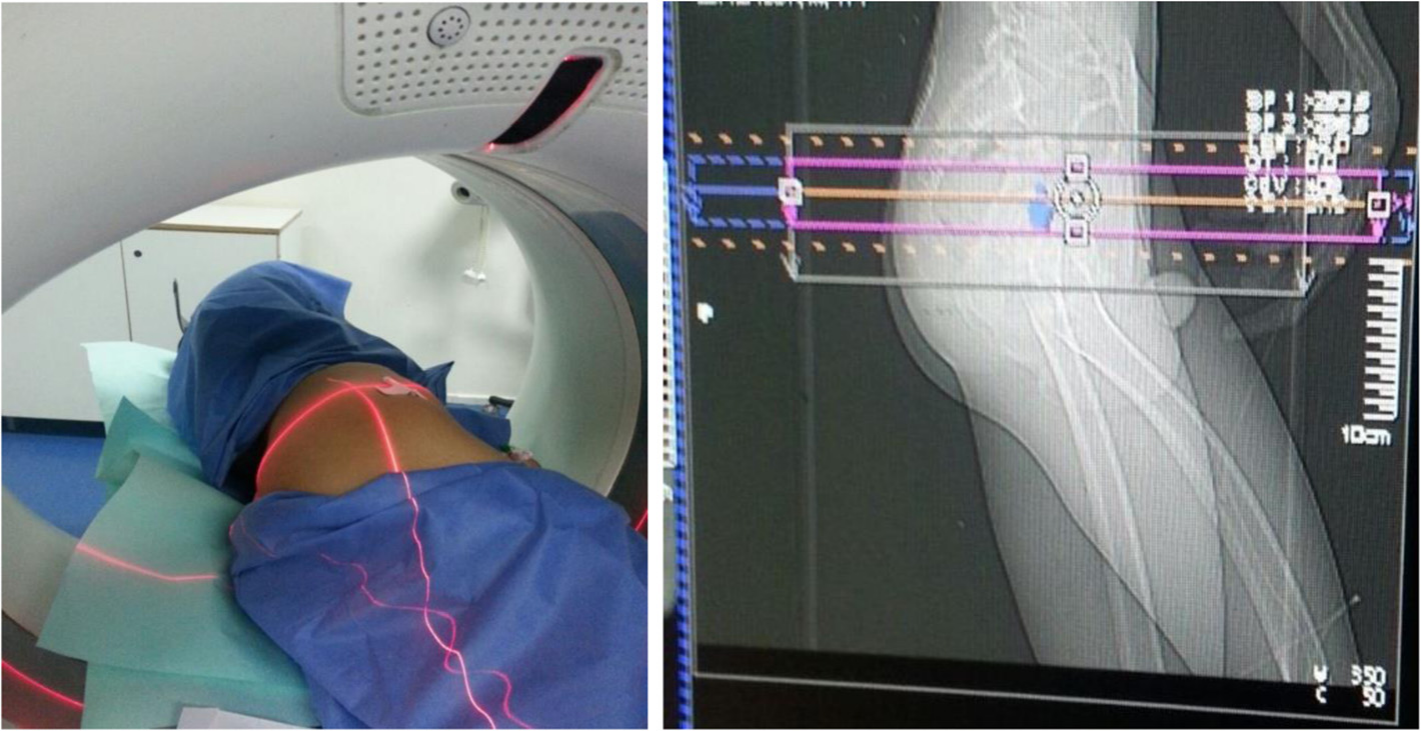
Locating osteoid osteoma by contiguous computed tomography slices
